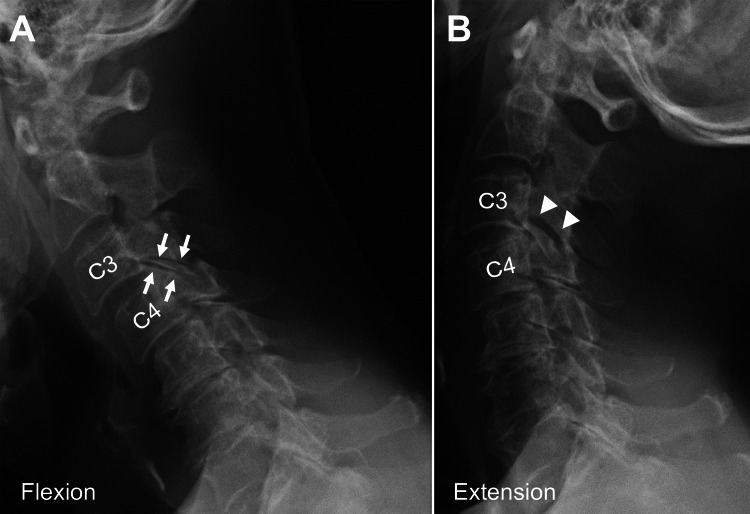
Preoperative lateral dynamic radiographs of the cervical spine. Flexion (A) and extension (B) radiographs reveal C3-4 horizontal translation as 2.5 mm and parallel shadows of the bilateral C3-4 facet joints during flexion (arrows), which enlarge markedly during extension (arrowheads).
